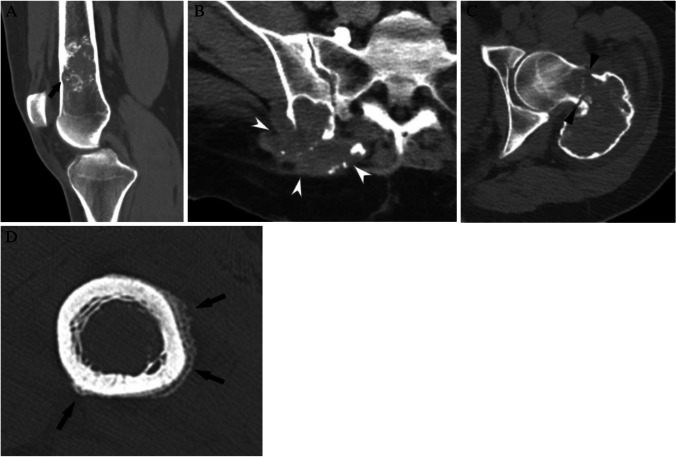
CT assessment of concerning features. A Coronal CT image shows cortical involvement (arrow) of the anterior femoral cortex from an enchondroma. B Axial soft tissue window CT image shows a destructive lung cancer metastasis in the ilium with large soft tissue component (pointed arrows). C Axial CT image shows a pathologic fracture (arrowheads) in the femoral neck due to a giant cell tumor of bone. D Axial CT image shows periosteal reaction (thin arrows) along the femoral cortex from presumed stress fracture
